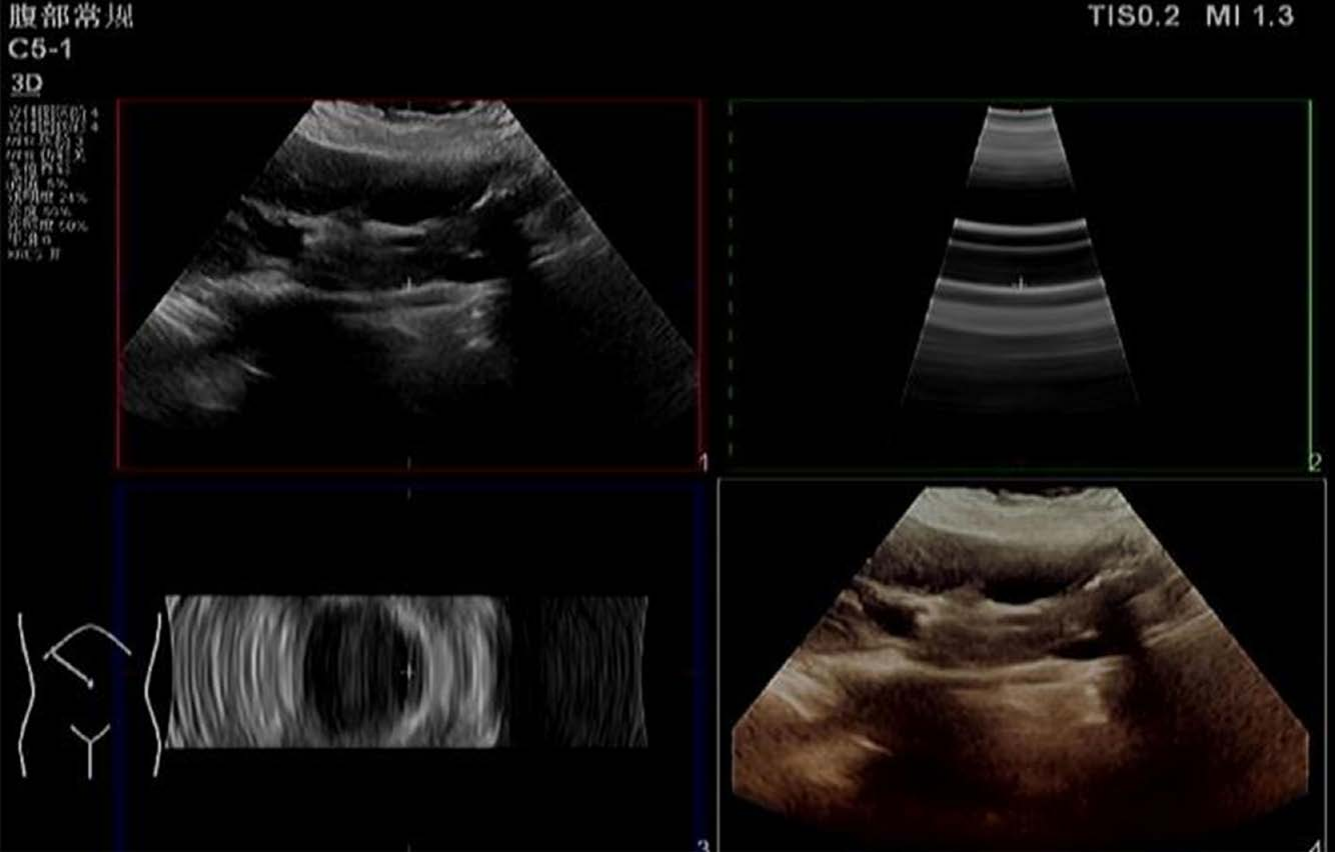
Ultrasound examination of the Liver. Liver ultrasonography revealed that the liver’s morphology and dimensions were within normal limits. However, the liver surface appeared irregular, and the liver edge was either sharp or blunt. The echotexture of the liver parenchyma was coarse. The hepatic vein was clearly visualized, although the vessel wall was irregular. There was no evidence of portal vein system dilation, and the intrahepatic blood flow was well visualized.
